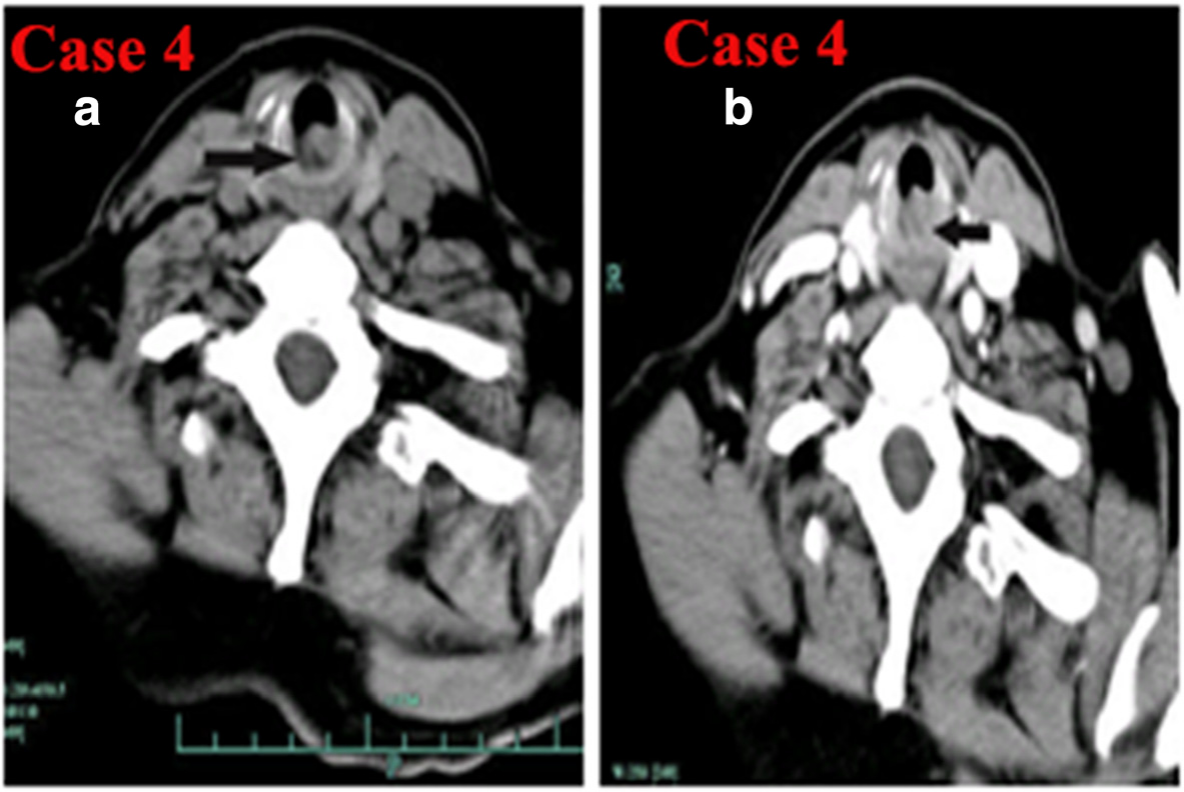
CT of laryngeal small-cell neuroendocrine carcinoma of Case 4. a) Noncontrast axial CT showing a heterogeneous well-defined soft-tissue mass in the subglottic regionnot involving the bilateral vocal cord and laryngeal cartilages. b) Contrast-enhanced CT showing that the lesion was mildly enhanced.
